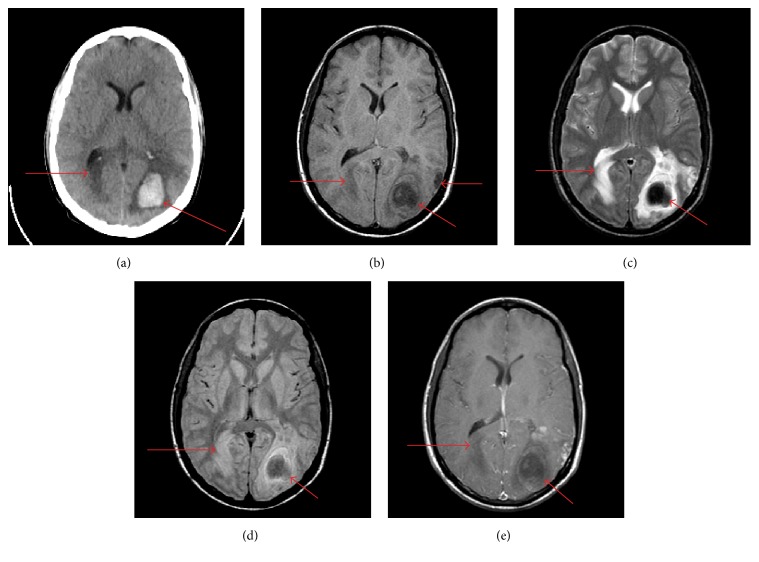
The clinical brain CT and MR images. (a) CT image, (b) MR-T1W image, (c) MR-T2W image, (d) MR-PDW image, and (e) MR-CE image.
